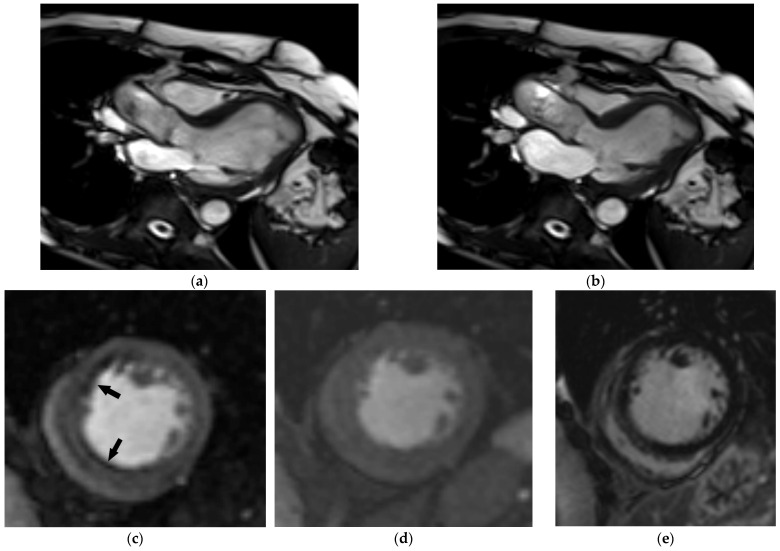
Comprehensive cardiac MRI in a 74-year-old female patient with a new onset of heart failure. End-diastolic (a) and end-systolic (b) 3-chamber view SSFP image depicting moderately impaired left ventricular systolic function (EF 31%). Midventricular short-axis adenosine-stress (c) and rest (d) perfusion image revealed stress-induced ischemia in the anterolateral and inferolateral wall (arrows) without evident late gadolinium enhancement (e). Coronary angiography chronic occlusion of the mid-LAD, as well as high-grade stenosis of mid-RCA and D1, were confirmed and treated.
